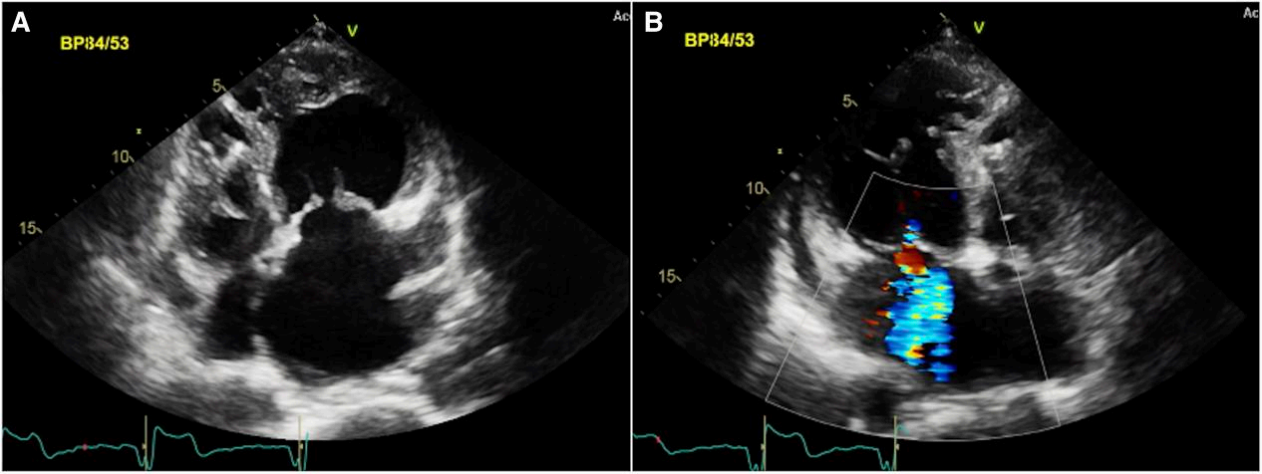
Transthoracic echocardiography of a 57-year-old male patient with ccTGA. *(A)*: apical four-chamber-view revealing the systemic RV with a hyper trabeculated apex in leftward position with accompanying moderate regurgitation of the systemic tricuspid atrioventricular valve in the apical two-chamber view *(B)*.
